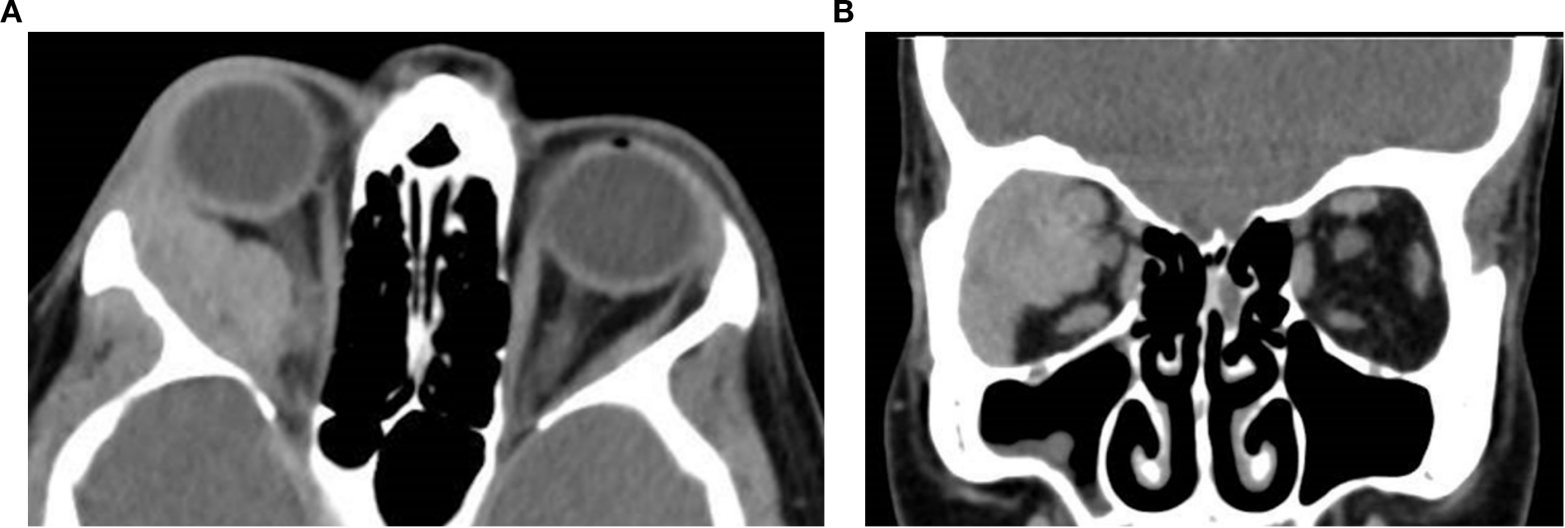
Axial (a) and coronal (b) post-contrast CT images of a 45-year-old female patient with adenoid cystic carcinoma of the right lacrimal gland. The images demonstrate an ill-defined, infiltrative soft tissue mass centered in the superolateral orbit, involving the lacrimal gland and extending into the extraconal space, with apparent displacement of the globe and the optic nerve. Subtle contour irregularity of the lateral orbital wall and zygoma was noted, with extension into the infratemporal fossa.
